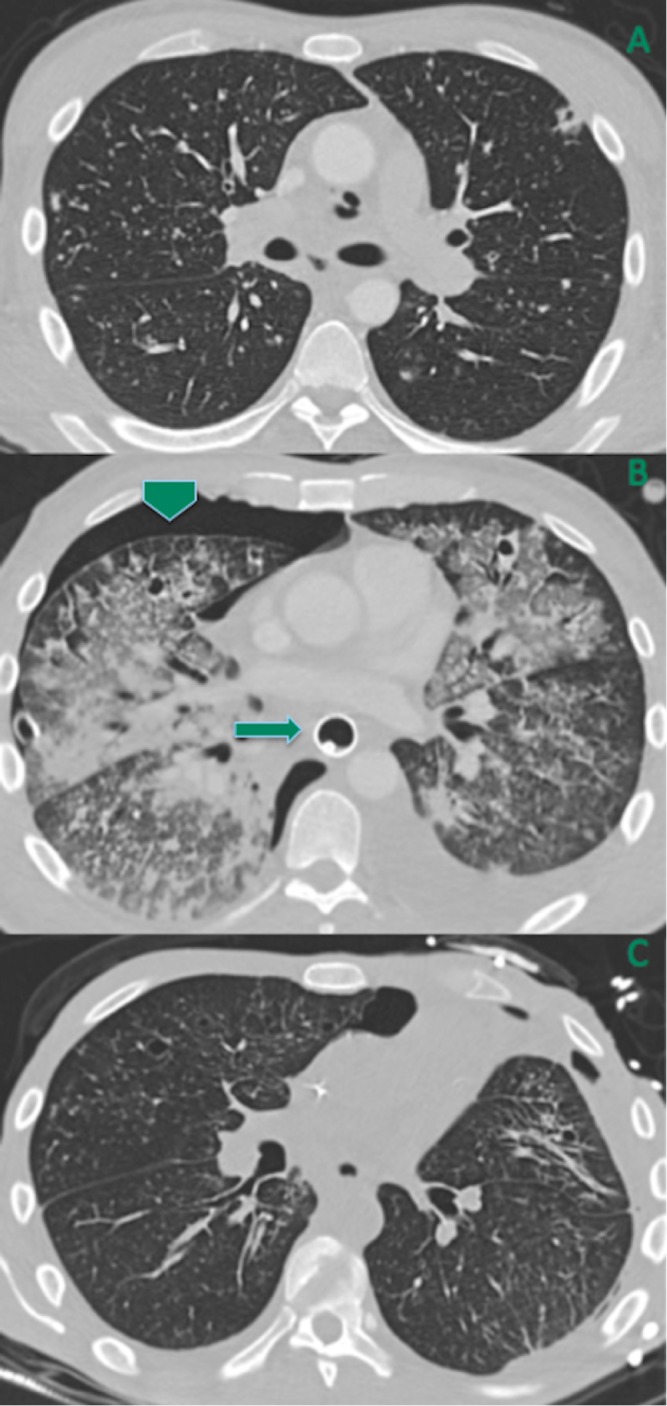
Sequential chest CT studies. (
